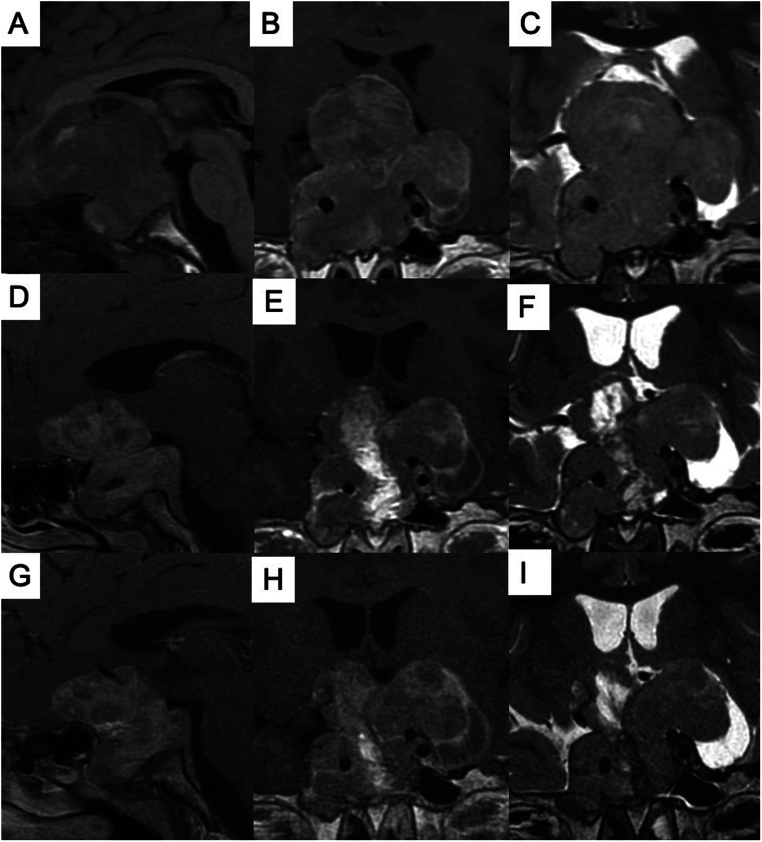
Magnetic resonance imaging. Sagittal T1 post-contrast (A), coronal T1 post-contrast (B), and coronal T2 (C) imaging prior to surgery demonstrate a 5.6 × 4.7 × 5.8 cm (transverse × anterior-posterior × craniocaudal) invasive sellar lesion (Knosp 4) with suprasellar extension, compressing the optic chiasm, isointense on T2 and with heterogeneous contrast enhancement, suggestive of cystic or necrotic degeneration. Sagittal T1 post-contrast (D), coronal T1 post-contrast (E), and coronal T2 (F) imaging after the two surgeries exhibit partial tumor resection. Sagittal T1 post-contrast (G), coronal T1 post-contrast (H), and coronal T2 (I) after 5 months of pasireotide and 2 months of cabergoline show no change in signal intensities or tumor shrinkage. The fat graft in the middle of the tumor reabsorbed partially.
